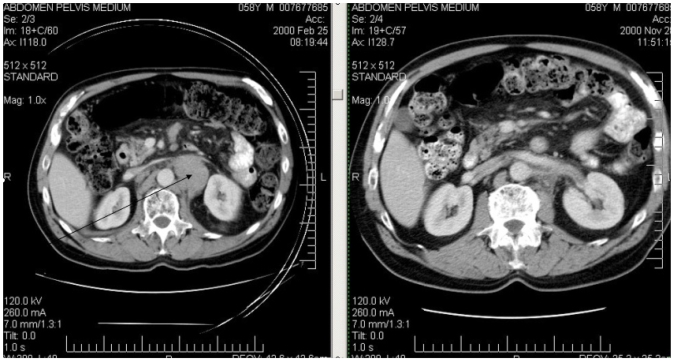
Patient 1: abdominal computed tomography scans showing regression of retroperitoneal adenopathy.
